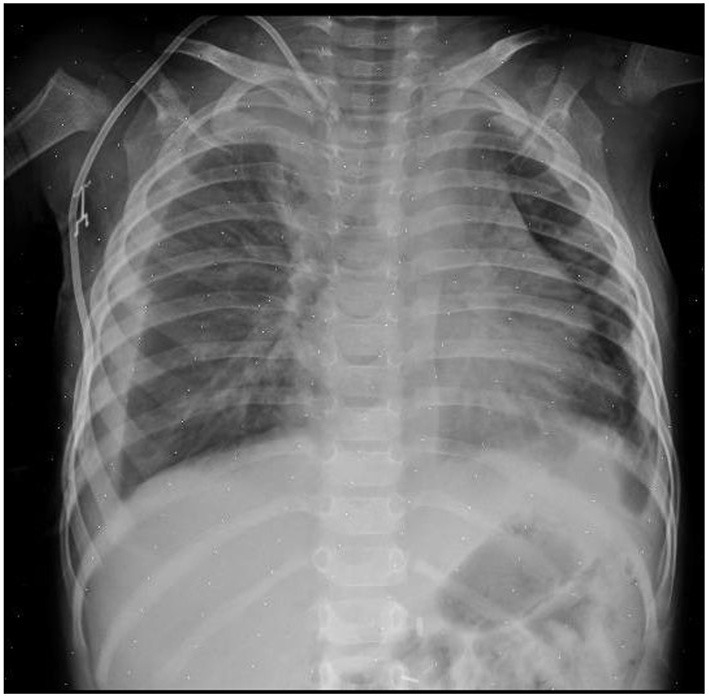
Chest x-ray showing dislocated tip of the catheter and bilateral pleural effusion.
